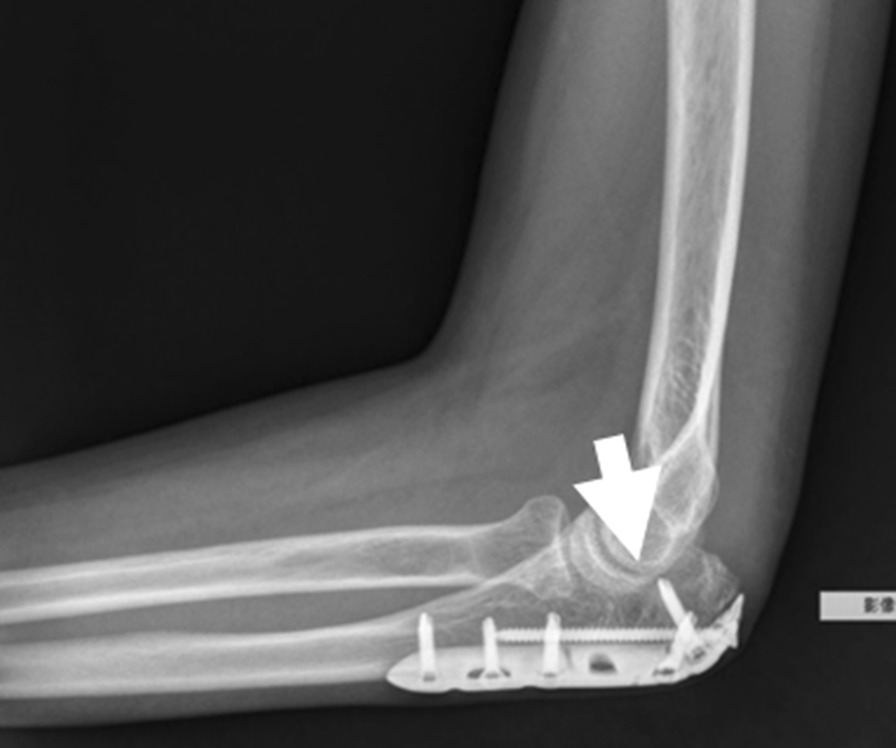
The X-ray-revealed fill-full of the nidus-like lesion (white arrow) in bone graft area demonstrates well healing of the bone
